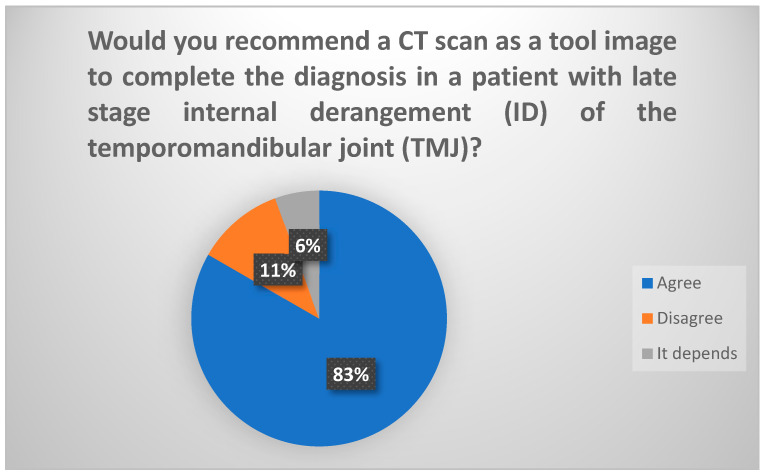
Delphi results regarding use of CT scan in late-stage ID of TMJ.
